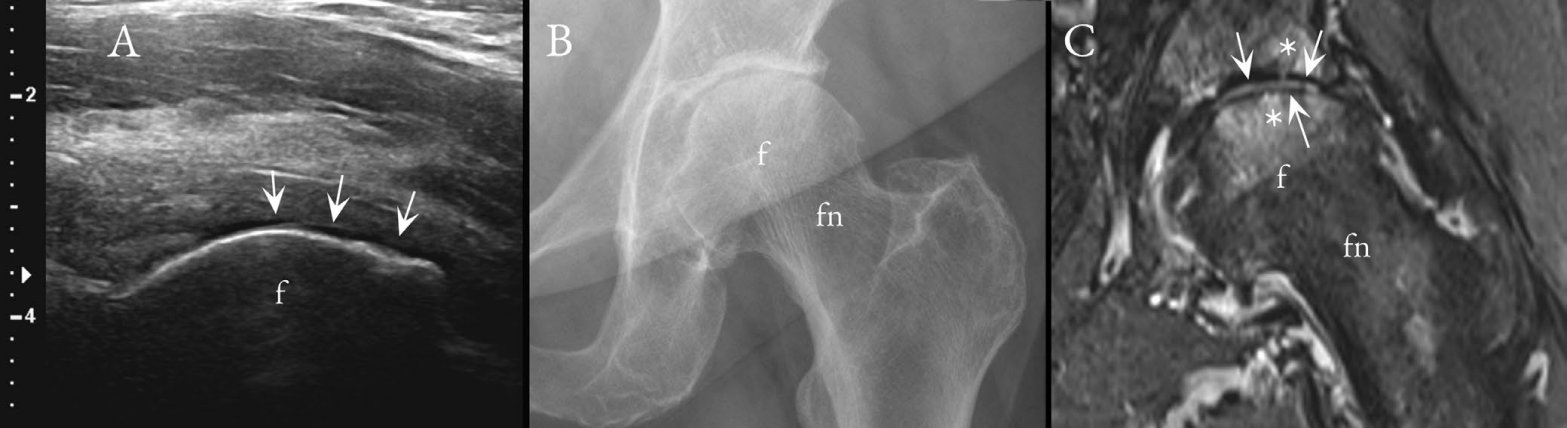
A 65‐year‐old woman with local wearing of the superolateral anterosuperior articular cartilage of the femoral head. In longitudinal US (A), cartilage wear is visualized as heterogenic intrachondral signal, cartilage thinning, varying cartilage thickness, and visualized local areas of cartilage defect. In this case, cartilage was evaluated as grade 2 (clear cartilage damage) in US. Anteroposterior CR (B) shows superolateral joint space narrowing. Coronal STIR MRI sequence (C) shows a large superior and superolateral cartilage defects and associated subchondral bone marrow lesion, indicative of full‐thickness cartilage damage. f, femoral head; fn, femoral neck.
